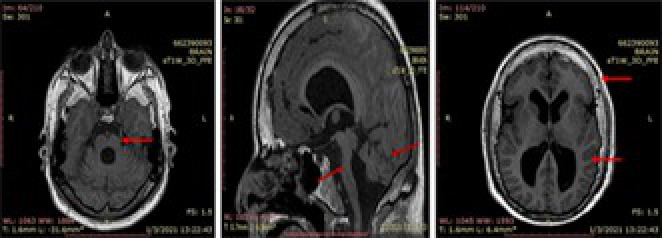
FIGURE 1 T1‐weighted MRI imaging of the brain showing brainstem and cerebellar hypoplasia, ventricular enlargement and lissencephaly predominantly involving the frontal lobes (red arrows).
